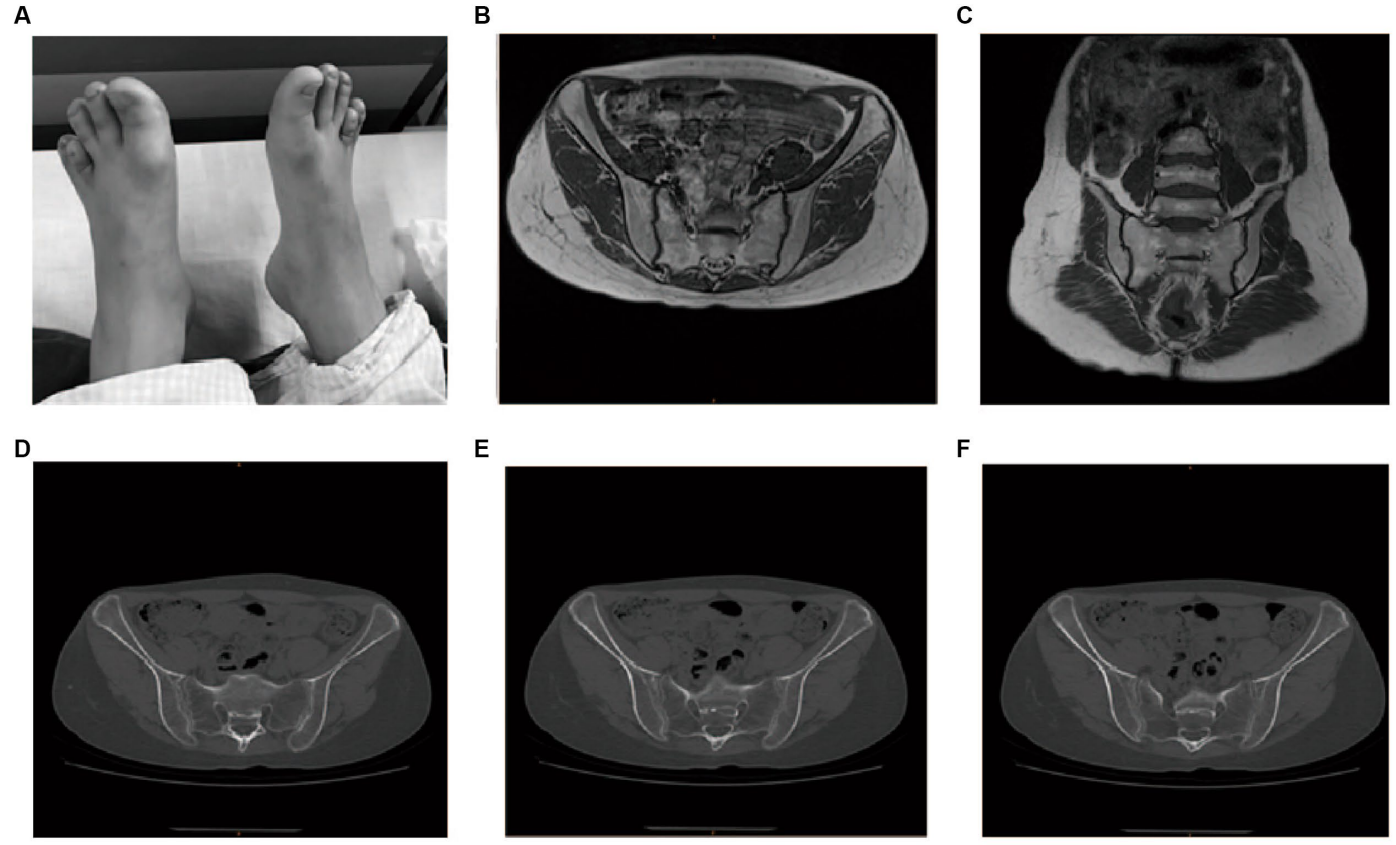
Physical examination, MRI, and CT imaging of the proband. (A) Bilateral short fourth metatarsal; (B,C) MRI for the sacroiliac joint; (D–F) CT for the sacroiliac joint.
